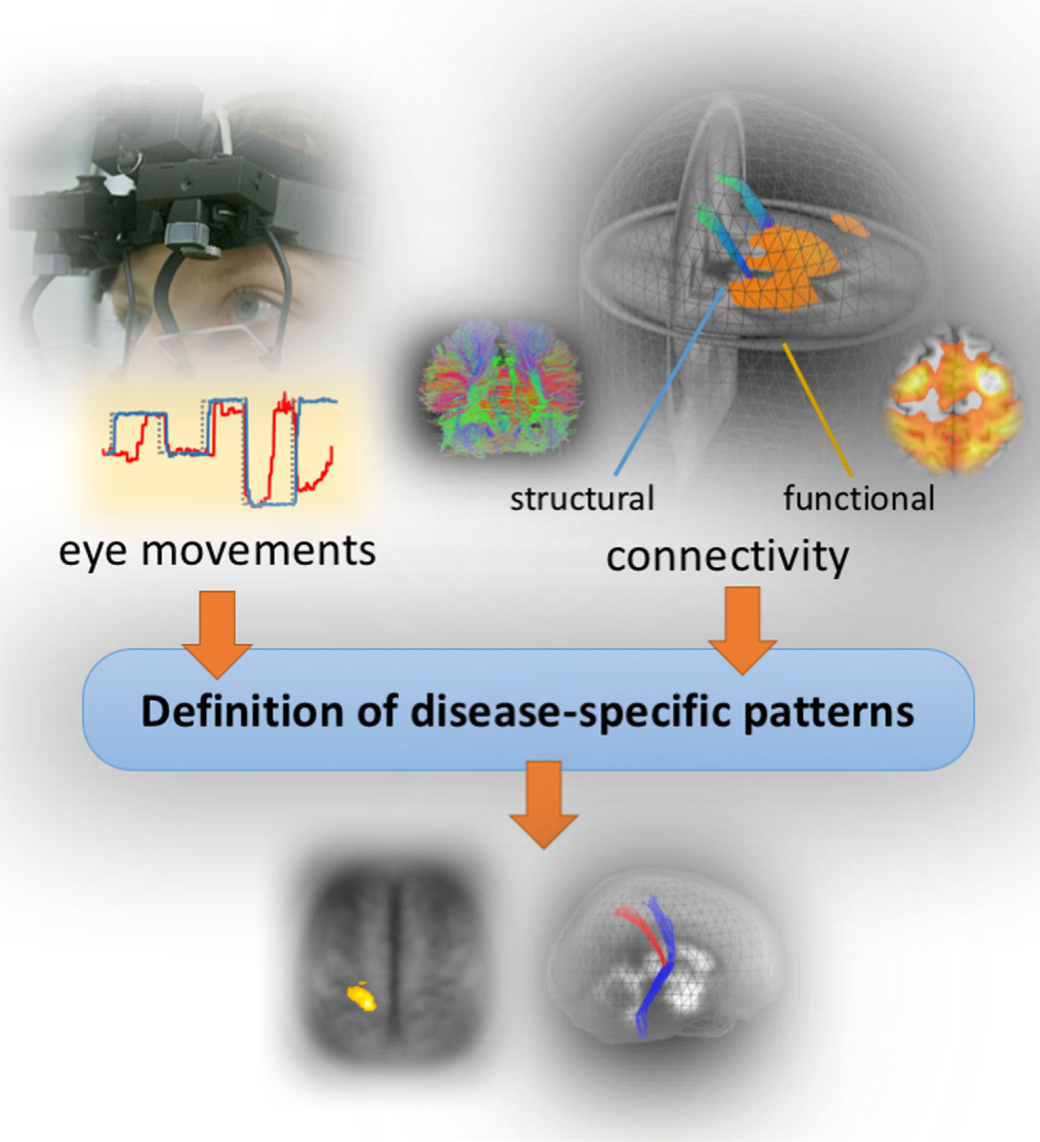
Concept of the bimodal study design. Structural and functional brain mapping of eye movement control by combining video-oculographically eye movements recordings (left upper panel) with structural and functional MR imaging data (right) in order to define disease-specific patterns.
